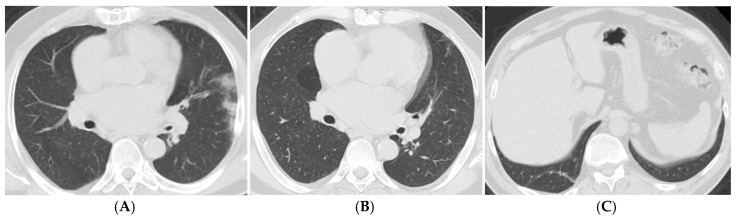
Axial CT scans of a 63-year-old male patient with mild to moderate COVID-19. (A) The CT obtained seven days after the onset of symptoms shows patchy, peripheral ground-glass opacities and consolidation areas bilaterally, with a CT severity score of 12. (B) The follow-up CT performed seven months later reveals the presence of air trapping in the medial segment of the right middle lobe, which was not observed in the previous examination. (C) In the right lower lobe, thick parenchymal bands and fibrotic densities are noted in the posterior basal segment.
